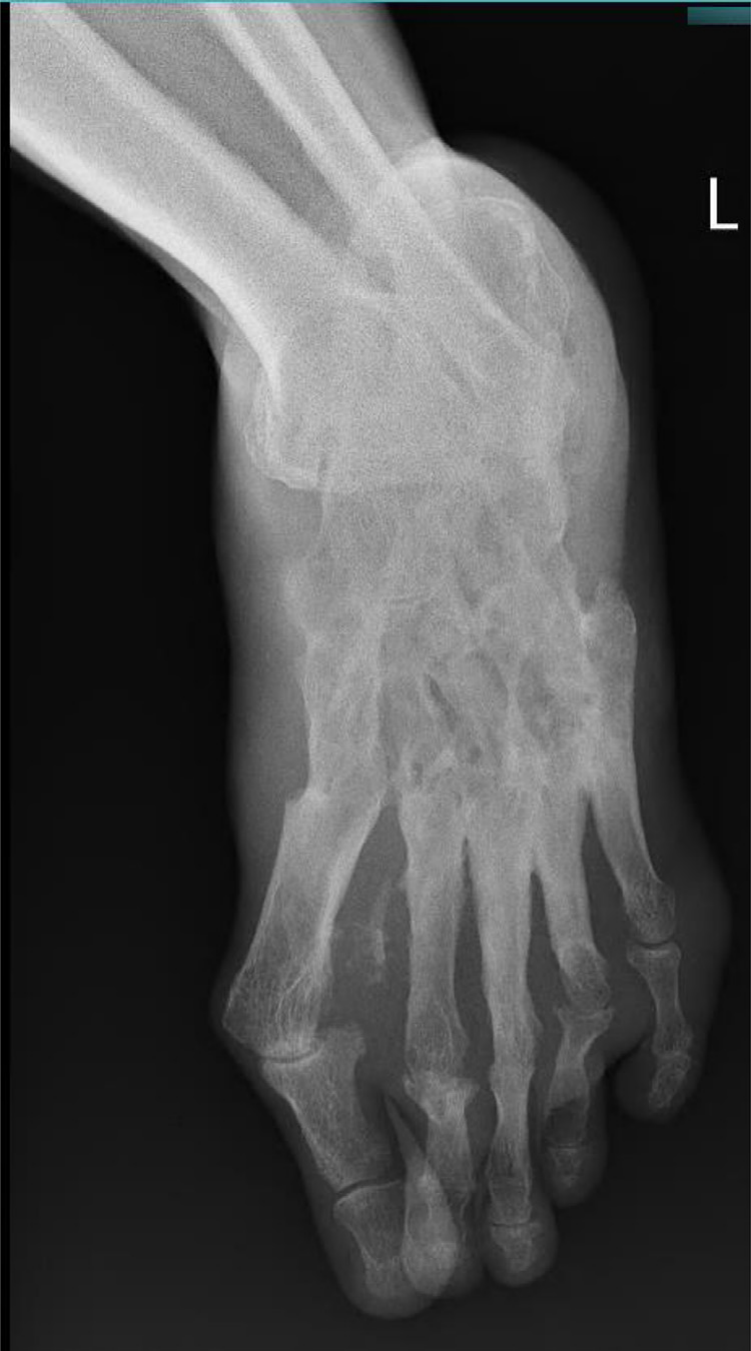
Radiograph foot (AP projection) showing ill-defined lytic and sclerotic lesions in tarsal bones with ankylosis at inter-tarsal and metatarsophalangeal joints.
